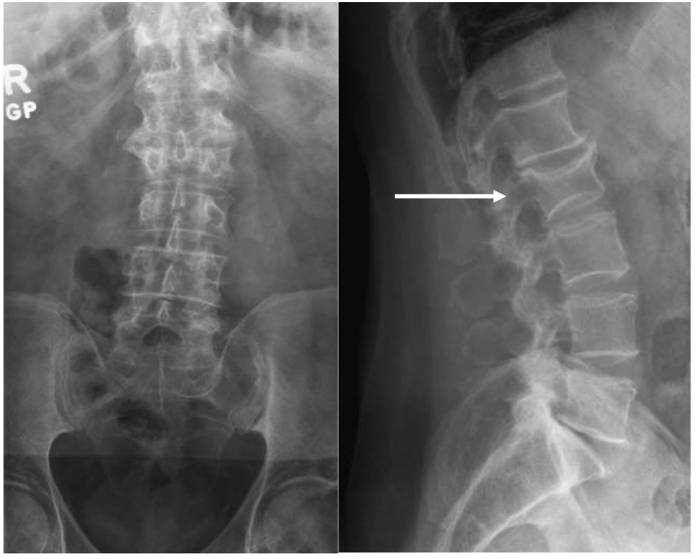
Radiography of the lumbar spine A) antero-posterior view B) lateral view. White arrow denotes compression fracture of L2.
